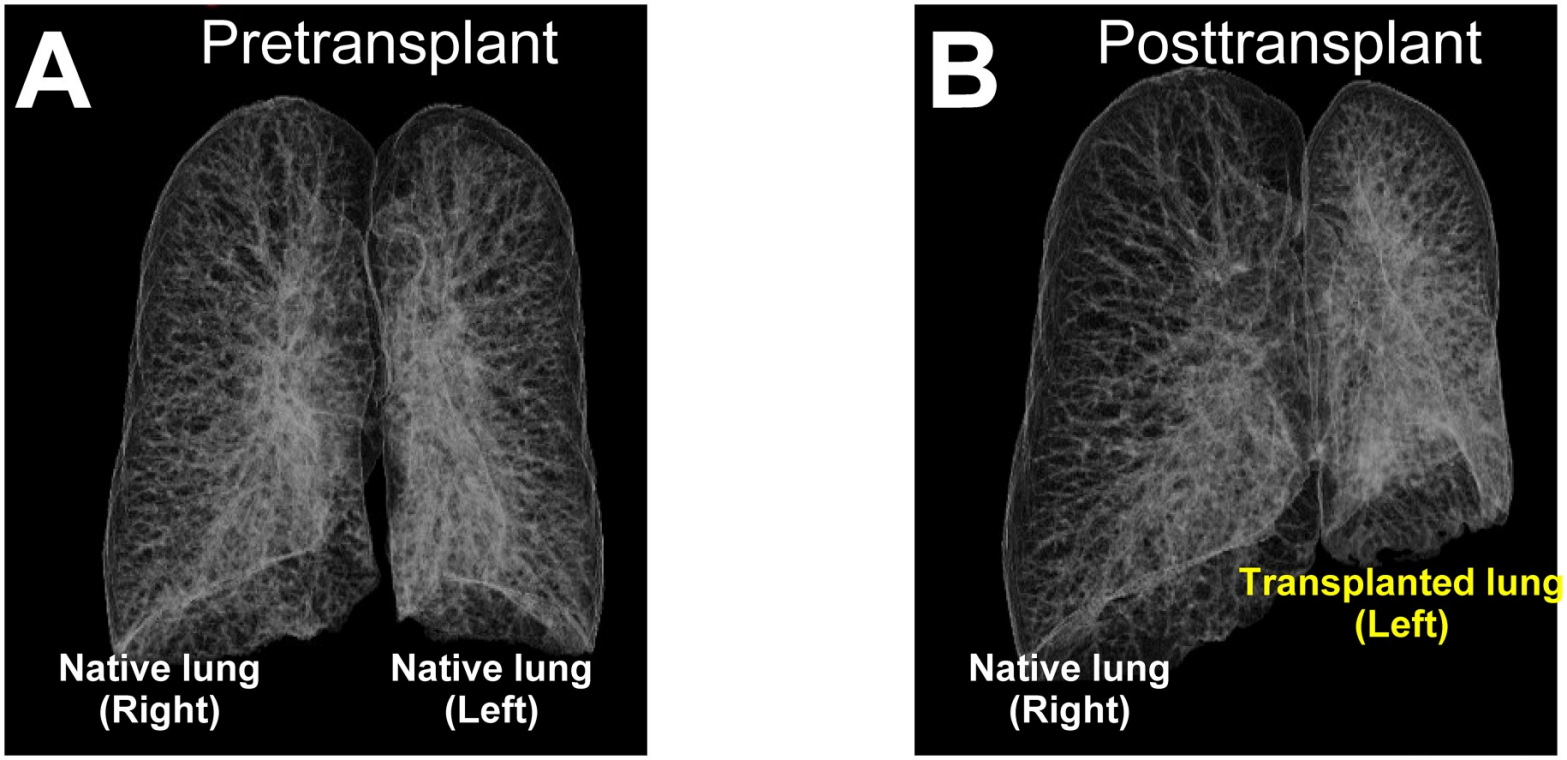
Pre- and post-transplant three-dimensional computed tomography (3D-CT) images of a case of single lung transplantation for lymphangioleiomyomatosis. (A) Pretransplant three-dimensional computed tomography (3D-CT) image of the lungs in a case of lymphangioleiomyomatosis. (B) Post-transplant 3D-CT images of the lungs in the case: a left single lung transplant case.
